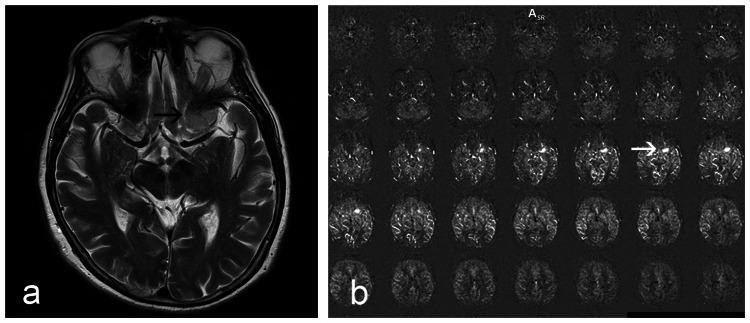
Meningioma around the left clinoid process. (a) T2-weighted image (black arrow). (b) Meningioma visible on ASL images (white arrow).
